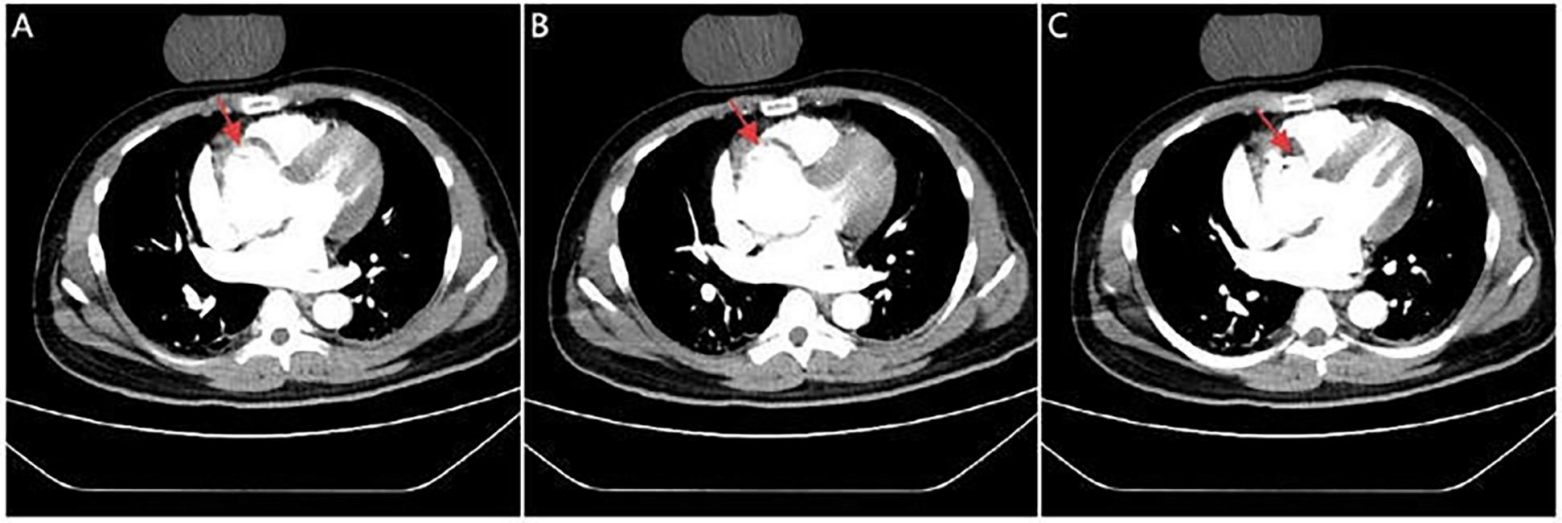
CTA images. Serial CTA images (A–C) show located right coronary sinus of valsava dissection (red arrow).
